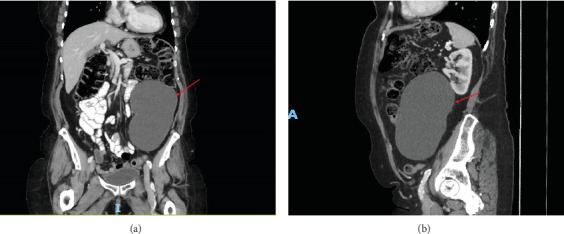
(a) Coronal view and (b) sagittal view of contrast-enhanced CT of the abdomen and pelvis showing a 9.4 × 16.1 × 10.6 cm cystic lesion within the left lower quadrant causing mild local mass effect.
